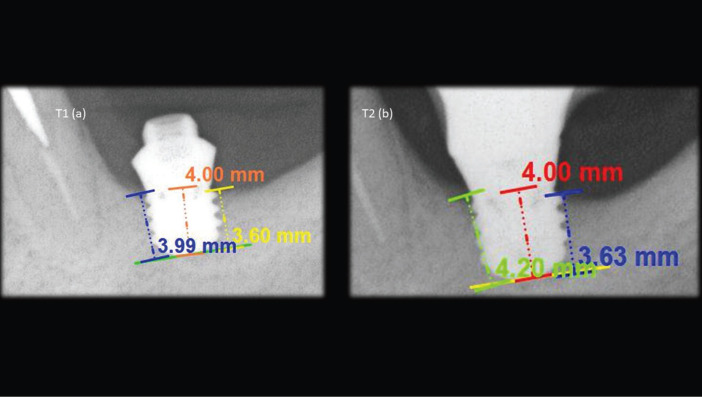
a) measurements made on the radiograph taken immediately after the implant installation; b) measurements made on radiograph after one year of implantation in masticatory function.
